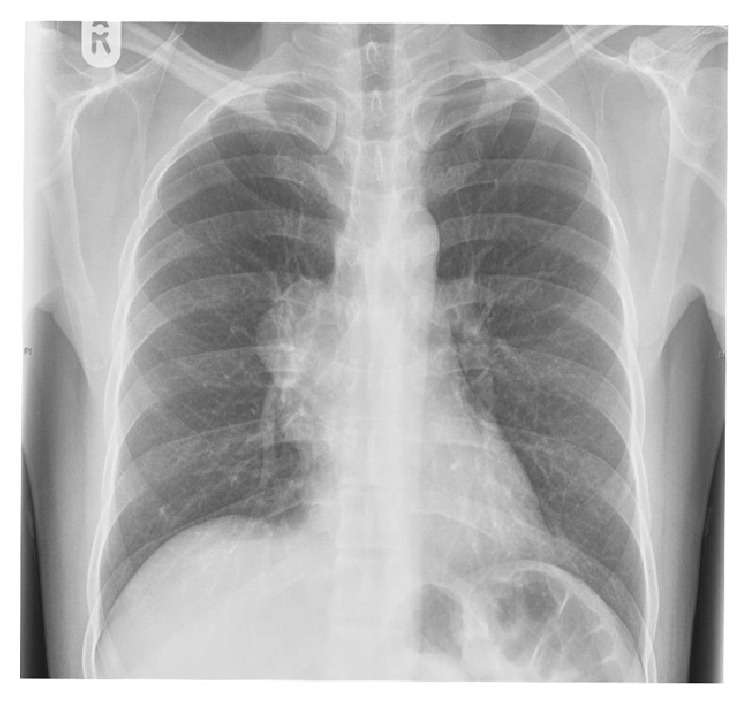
Chest X-ray showing right sided hilar mass.
